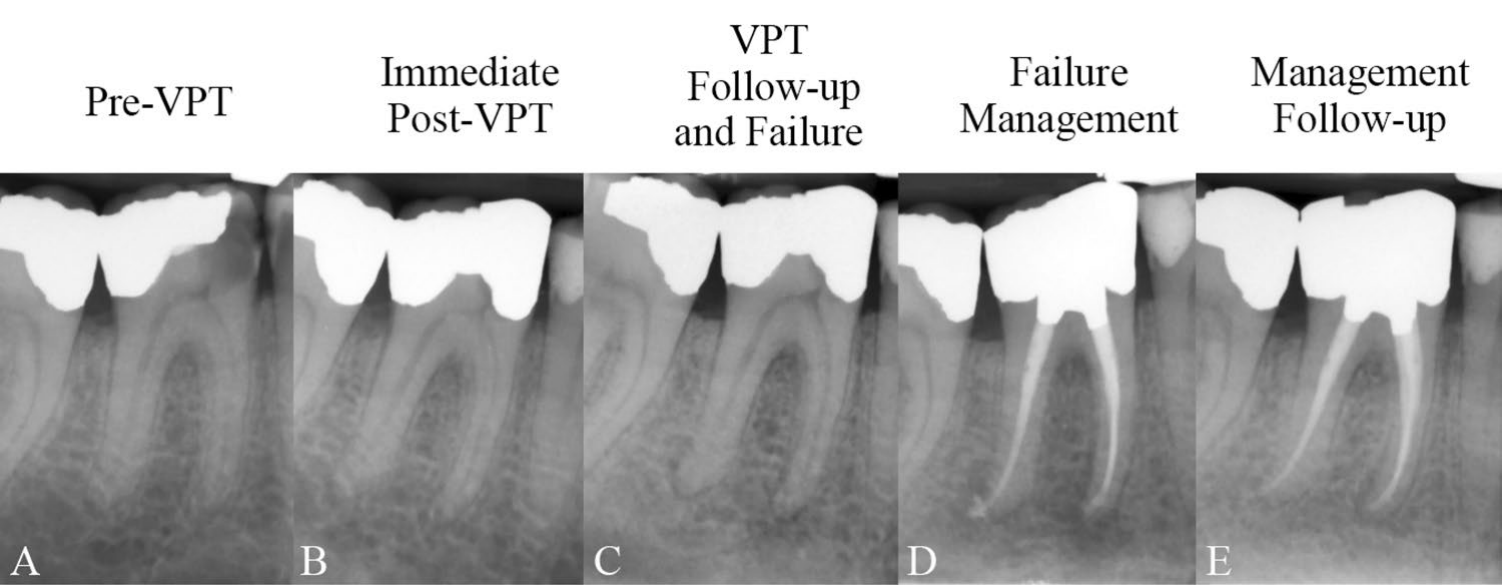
(A) Carious lesion on the lower right first molar of a 41-year-old man diagnosed with irreversible pulpitis; (B) Direct pulp capping and amalgam filling carried out; (C) Symptomatic tooth with periapical periodontitis evident at 6-month follow-up, confirming failure; (D) Root canal treatment and amalgam build-up of the tooth completed in a single session; (E) Healed periapical lesion and functional tooth identified at 44-month follow-up, confirming success.
